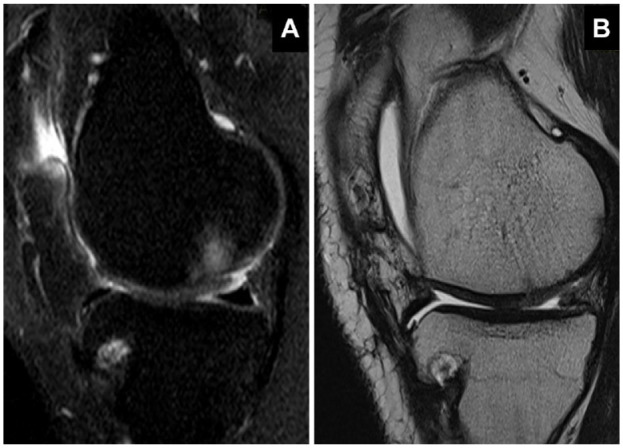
MRI imaging of a Chondro+-treated subject knee before scaffold implantation (A) and at 7 months of follow-up (B). (A) Sagittal proton density-weighted fat-saturated (PDW-FS) image showing the focal chondral defect of the Medial Femoral Condyle (MFC) with thinning of the cartilage. (B) Sagittal T2-TSE (turbo-spin-echo) image showing the regenerated cartilage tissue with focal hyperintense area at 7 months after Chondro+ implantation. MRI = magnetic resonance imaging.
